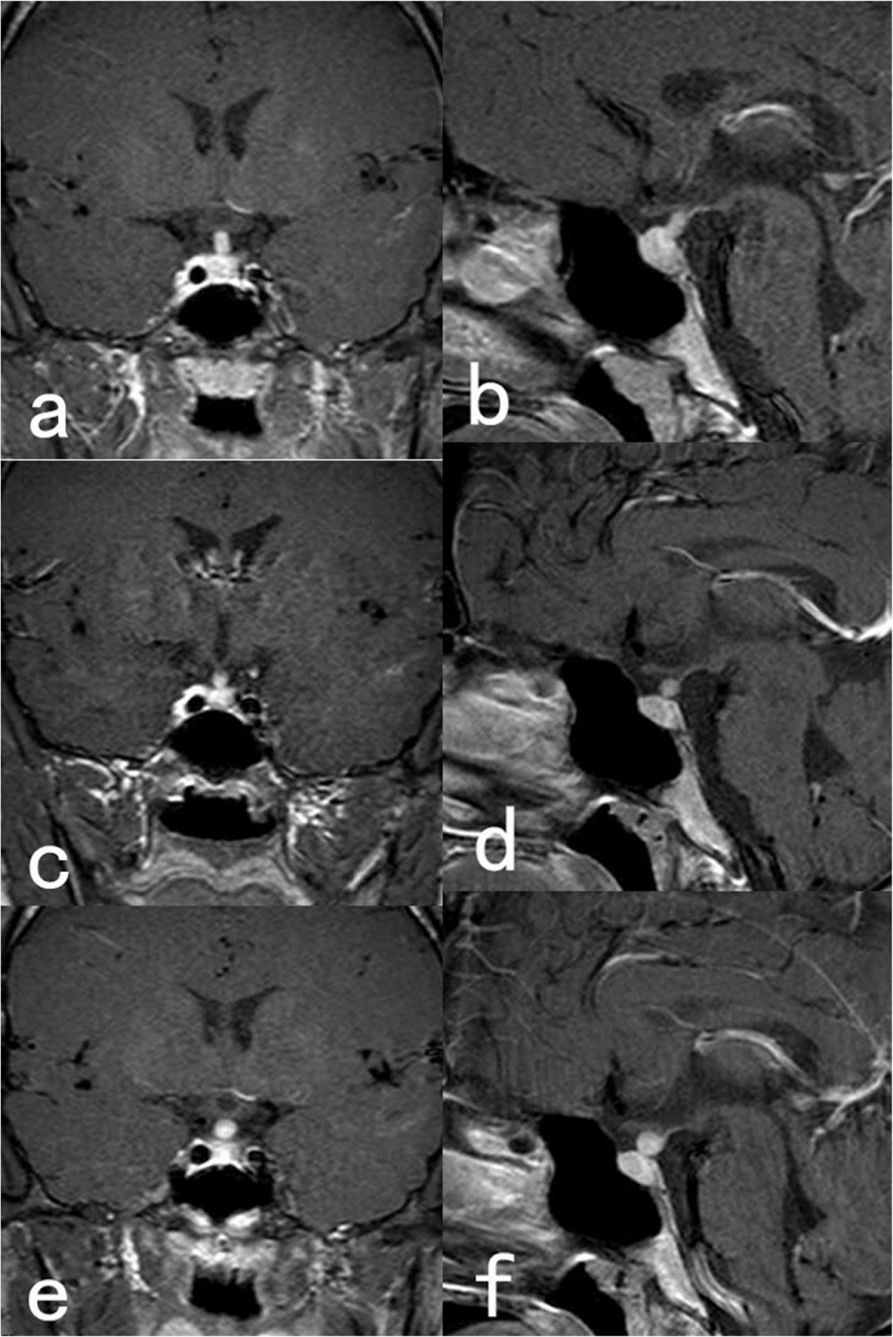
a Coronal and b sagittal magnetic resonance imaging (MRI) (T1-weighted with gadolinium enhancement) demonstrating an enlarged pituitary stalk and the neurohypophysis hypersignal deficiency. c Coronal and d sagittal T1WI showing the pituitary stalk is a nodular thickening with both size and shape have been changed after the patient discontinued the treatment for 1 month. e Coronal and f sagittal T1WI showing the nodular-thickening pituitary stalk is continuous enlarging after the patient continued the oral drugs for 2 months
